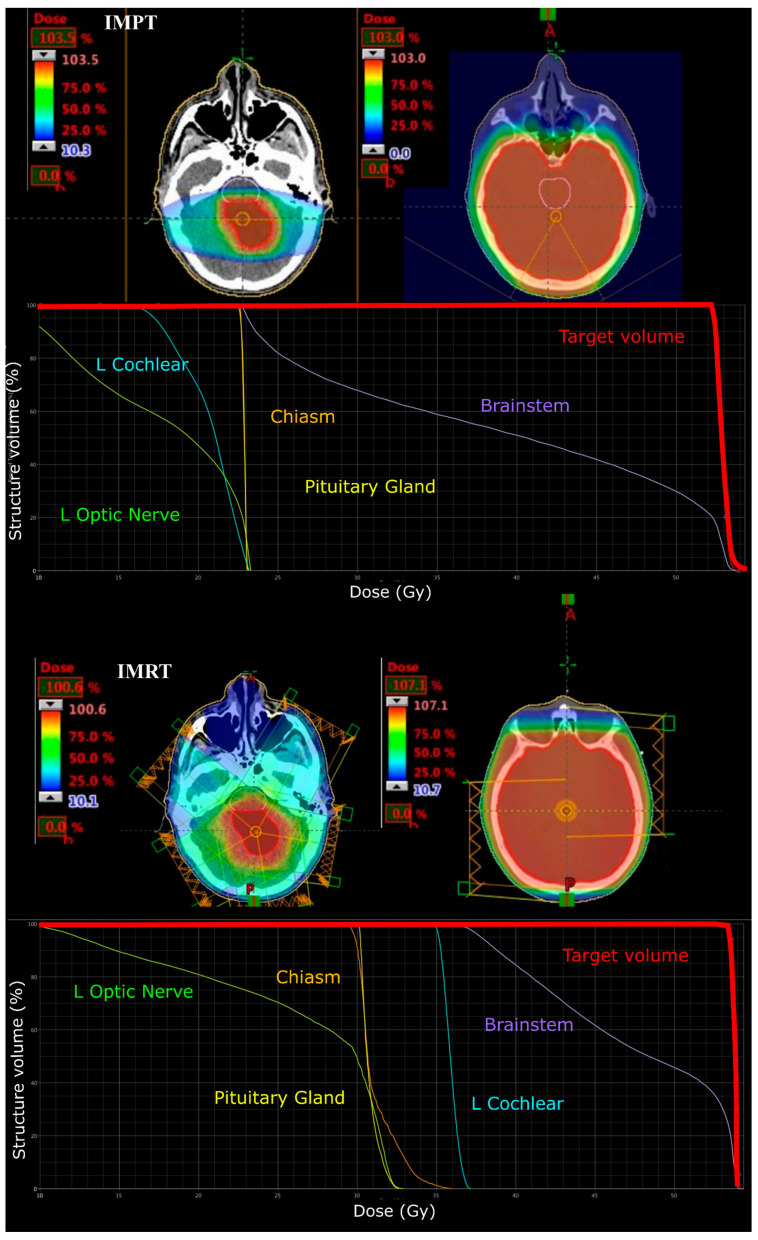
Colourwash dose distributions and dose–volume histograms for a 13-year-old male with infratentorial medulloblastoma. Typical planning beam arrangement viewed on axial CT images for IMPT tumour bed (top left) and craniospinal (top right) and IMRT tumour bed (bottom left) and craniospinal (bottom right) plans. Respective dose–volume histograms are shown below.
